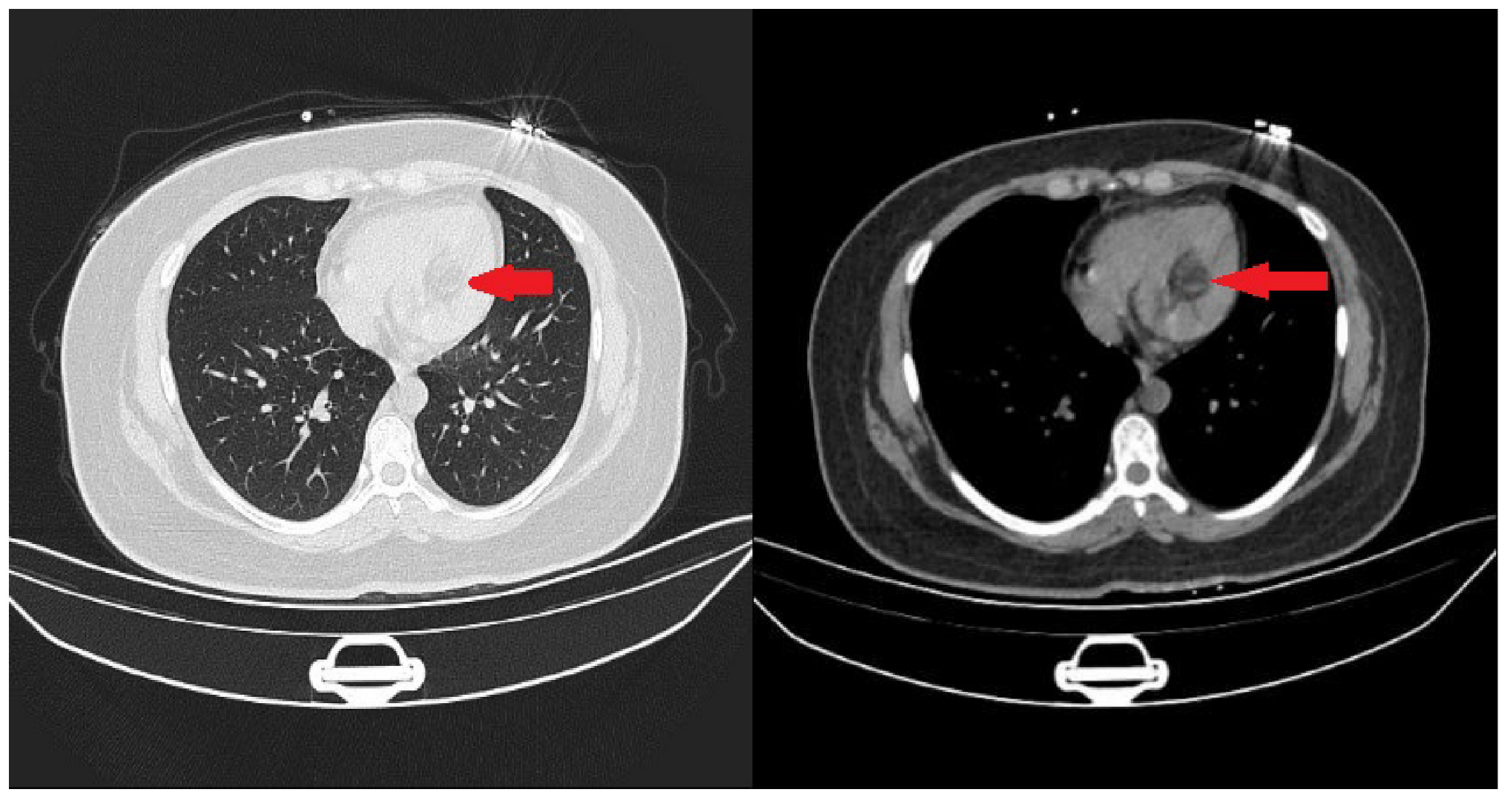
Chest computed tomography scan in the left ventricle. The arrow indicates a tumor in the left ventricle.
